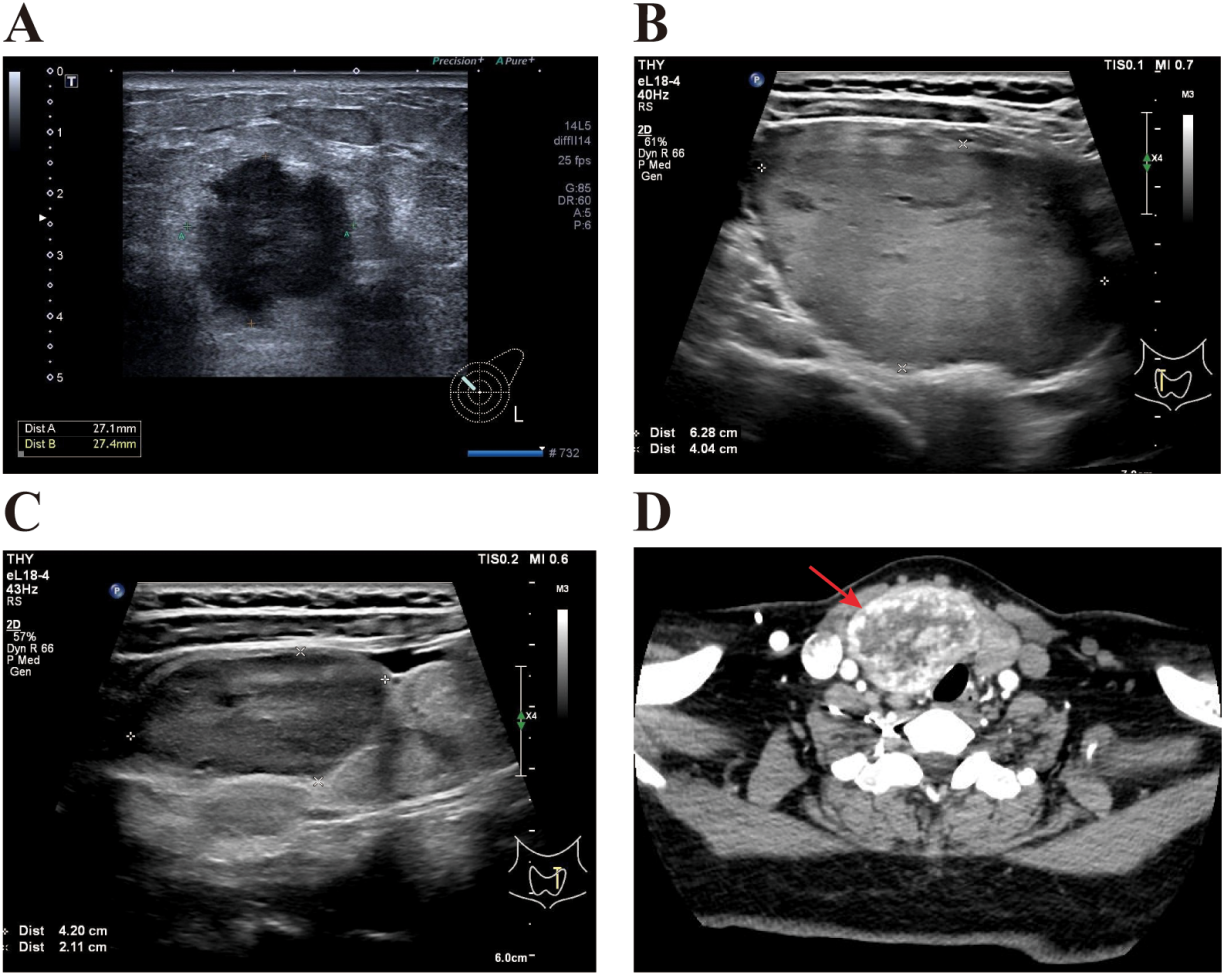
Preoperative ultrasound and computed tomography (CT) images of the breast and thyroid. (A) Doppler ultrasound of the breast and axilla shows a mass at the 10 o’clock position of the left breast, measuring 2.7×2.7 cm. The mass is highly suspicious for ductal carcinoma *in situ*, BI-RADS category 4c. (B, C) Doppler ultrasound of the thyroid and neck reveals multiple nodules in both thyroid lobes, with the largest on the right side measuring approximately 6.3×4.0 cm and on the left side measuring approximately 4.2×2.1 cm, both classified as TI-RADS 4a. (D) Thyroid CT scan shows a large mass on the right thyroid lobe compressing the trachea.
